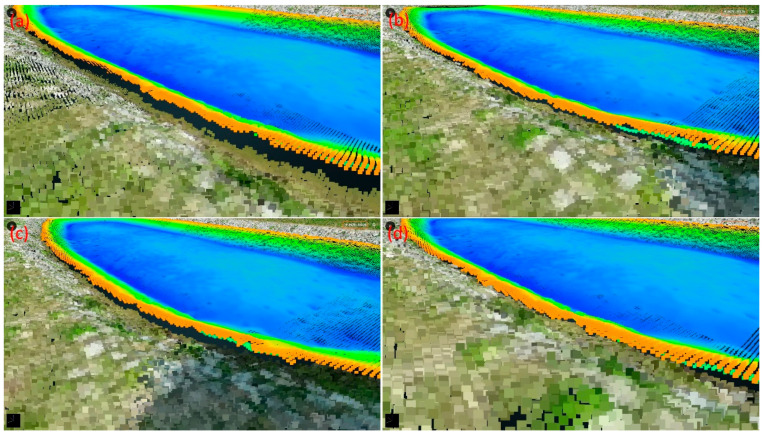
The partial fusion results of USV-UAV point clouds in study area 1: (a) without the CT method, (b) with the 4-parameter CT method, (c) with the 6-parameter CT method, and (d) with the 7-parameter CT method. The entire point cloud fusion results for (a–d) can be found at the following four links (accessed on 30 August 2024): https://www.pointbox.xyz/clouds/66b32be2817a42b2255567e2, https://www.pointbox.xyz/clouds/66b3257c817a4296c65567da, https://www.pointbox.xyz/clouds/66b328eb817a4256e35567de, https://www.pointbox.xyz/clouds/66b32b84817a42e34f5567e0.
